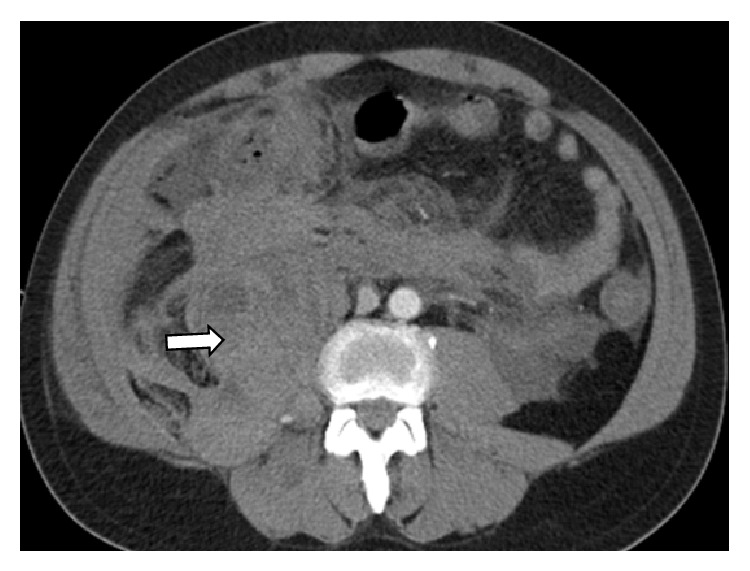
Stat CT abdomen/pelvis. Interval development of a large retroperitoneal hemorrhage centered around the right psoas muscle, with extension to the right spinous erector and iliacus musculature (see arrow).
